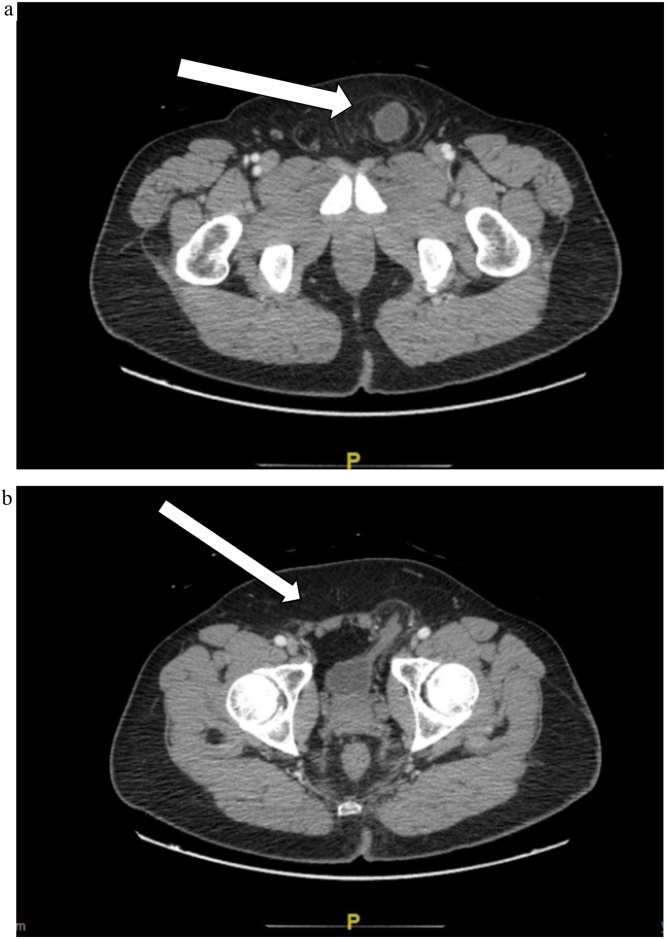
A. CT scan showing bladder herniated/protruded into the Left groin; axial view. B. CT scan of the pelvis shows a slice through the bladder intraperitoneally and a part of the bladder herniating through the Left inguinal hernia/groin. The urinary bladder is stretched into the left inguinal hernia. No bladder mass or calcifications seen/present.
